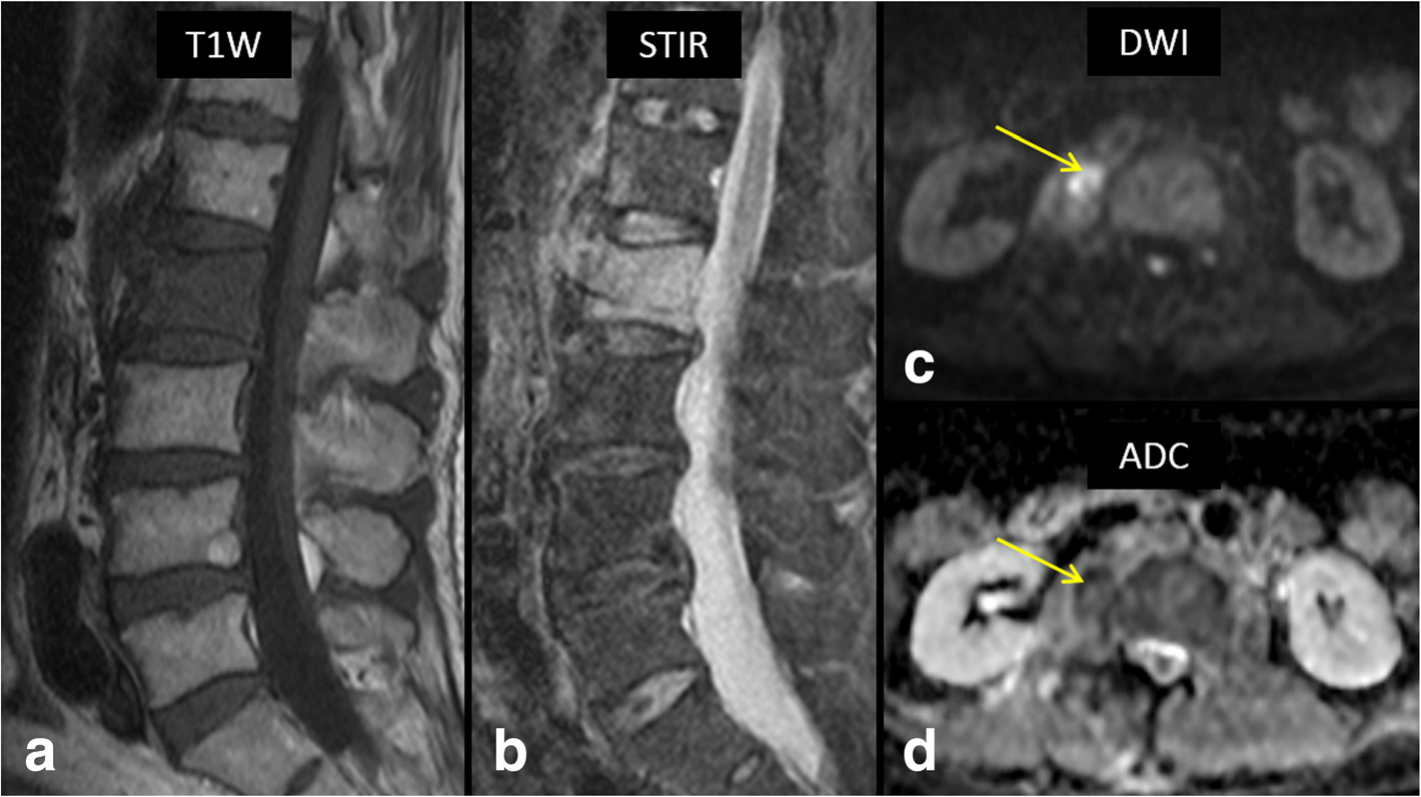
64-year-old male with fever and low back pain. Sagittal T1 W (a) and STIR (b) MR images show extensive bone marrow edema involving entire L2 vertebral body and anterior portion of L1 vertebral body. High b-value (1000) axial diffusion weighted image (c) shows a right paraspinal soft tissue lesion with restricted diffusion (*arrow*) with associated decreased ADC values on ADC map (d, *arrow*). Biopsy of the lesion confirmed bacterial spondylodiscitis at L1/2 with a paraspinal abscess. *Staphylococcus aureus* was the causative organism
